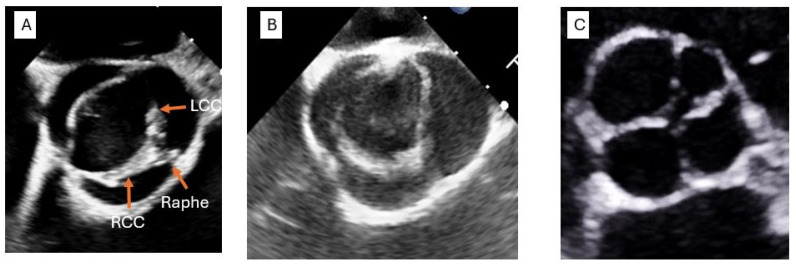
Examples of non-tricuspid aortic valve morphologies on echocardiography. (A) Short-axis view of the AV by TEE showing BAV with a raphe between the RCC and LCC. (B) Short-axis view of the AV by TEE of a UAV. (C) TTE image of a Type C QAV characterized by two larger equally sized cusps and two smaller equally sized cusps. RCC: Right Coronary Cusp; LCC: Left Coronary Cusp.
